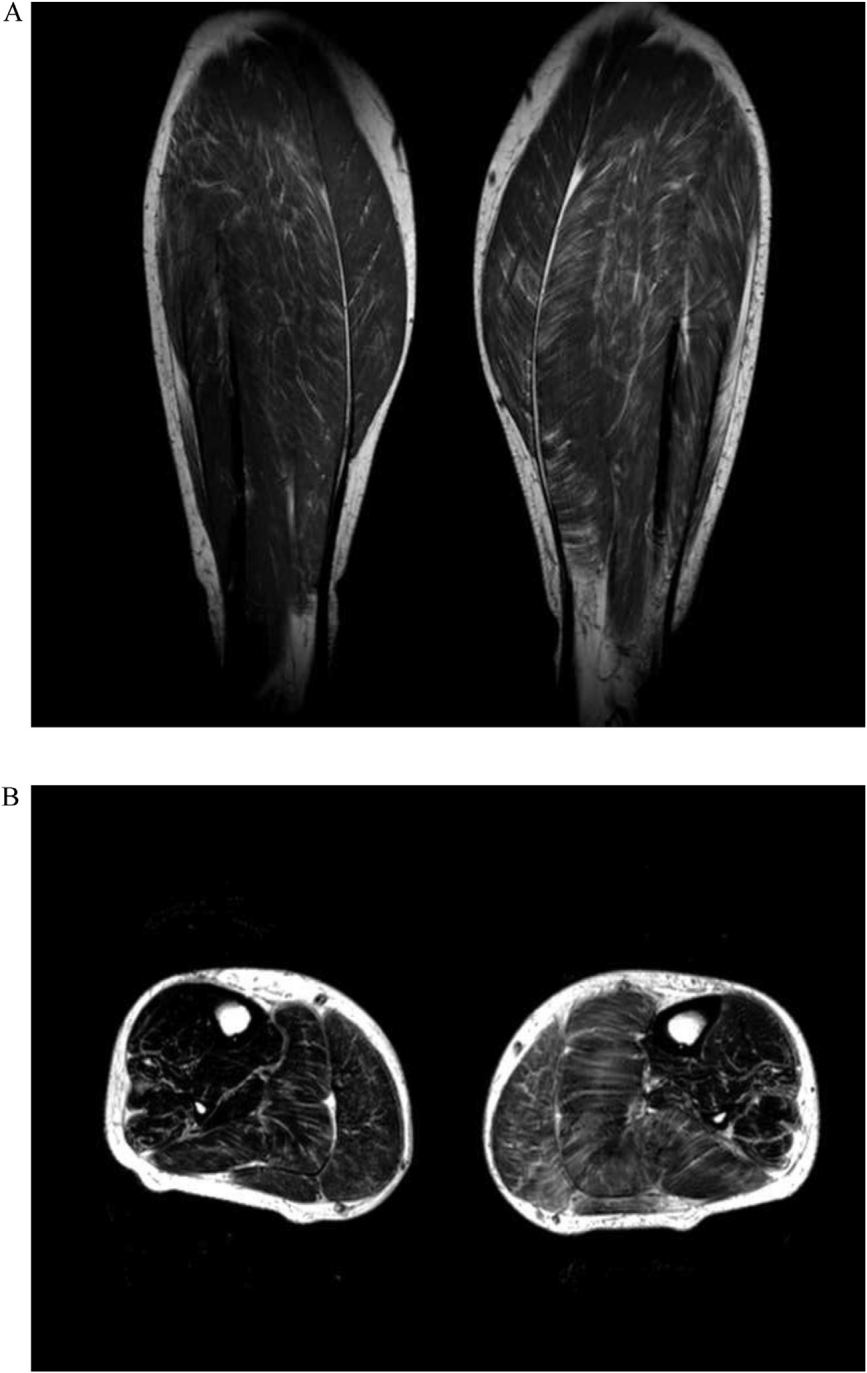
Coronal T1-weighted (Fig. 1A) and Axial T2-weighted (Fig.1B) images of the calves demonstrating asymmetric enlargement of the musculature of the left calf with evidence of fatty infiltration.
